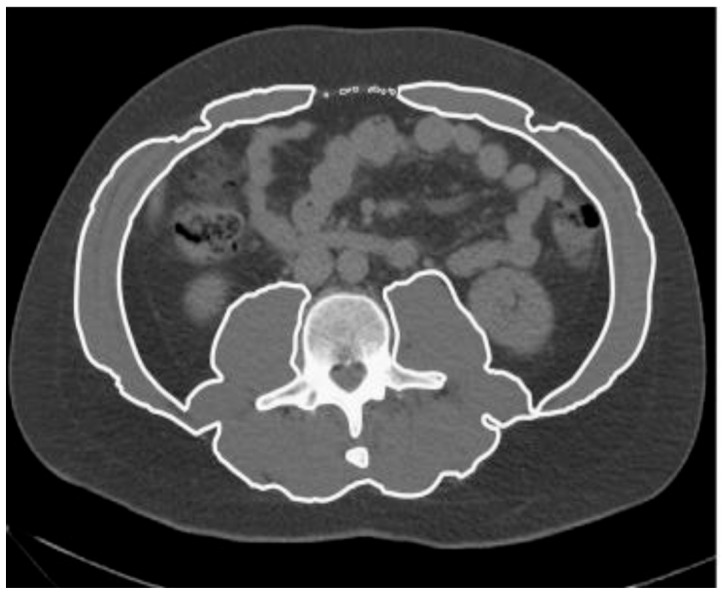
CT scan of a patient from our series showing the method for evaluating sarcopenia and myosteatosis: the slice considered corresponds to the level of the third lumbar vertebra; the skeletal muscle area is obtained from the combination of the psoas, paraspinal, and abdominal wall muscle areas.
